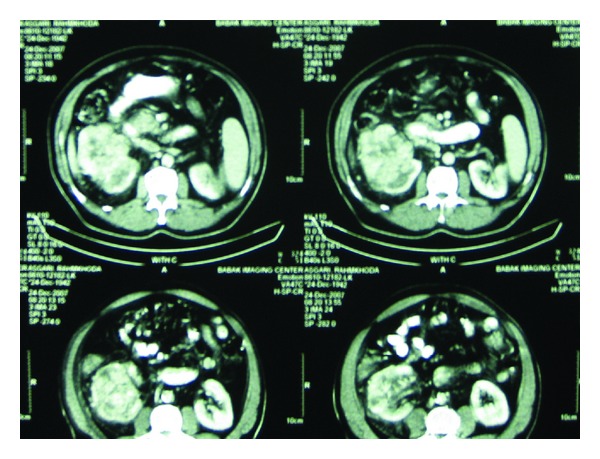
Contrast enhanced abdominal CT scan revealing the first tumor of the patient, right RCC.
